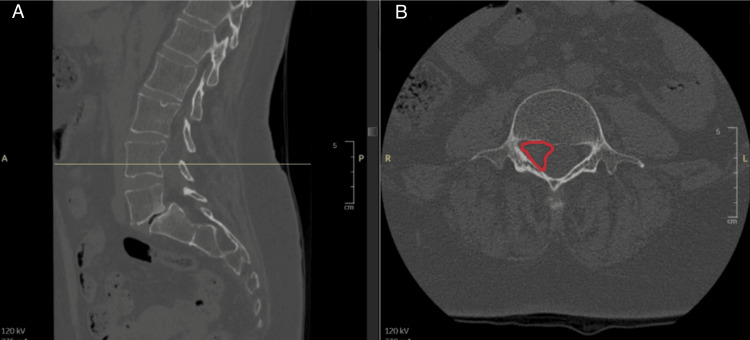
Lumbosacral CT scan demonstrating an intradural extramedullary lesion in the L4-L5 region (A) Sagittal CT (bone window) with the reference line indicating the level of the axial image. (B) Axial CT (bone window) at the L4 vertebral body level showing an intradural extramedullary lesion (highlighted) CT: computed tomography
